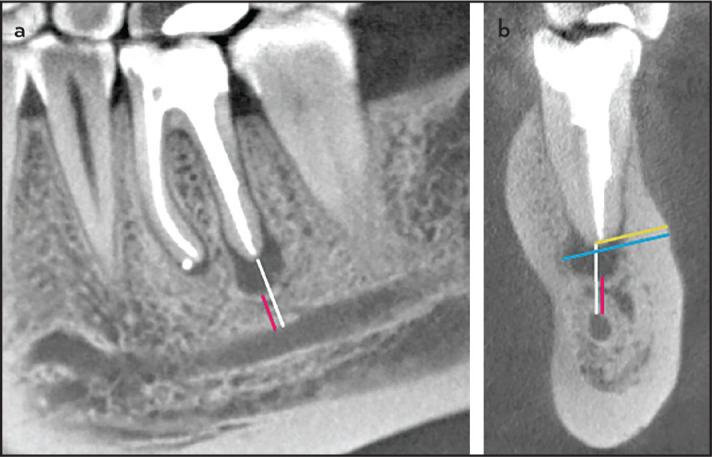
CBCT scans of the lower left first molar in a 34-year-old female. (a) Sagittal cut showing the shortest distances from the distal root apex (white line) as well as from the inferior margin of the lesion to the roof of the mandibular canal (red line). (b) Coronal cut exhibiting the same distances, but also the shortest distances from the buccal bone surface to the apex (yellow line) as well as to the lingual margin of the lesion (blue line) CBCT: Cone-beam computed tomography
